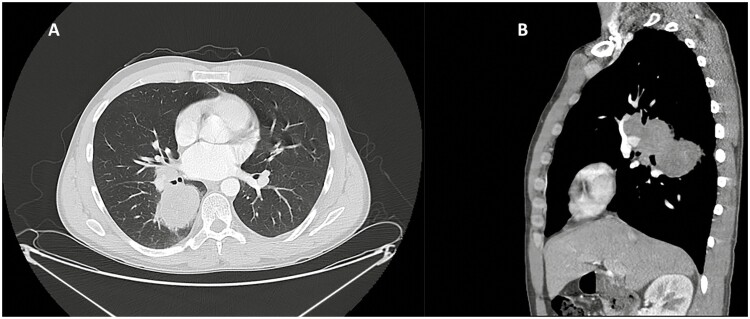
CT scan of chest before surgery. (A) Axial view and (B) sagittal view.
